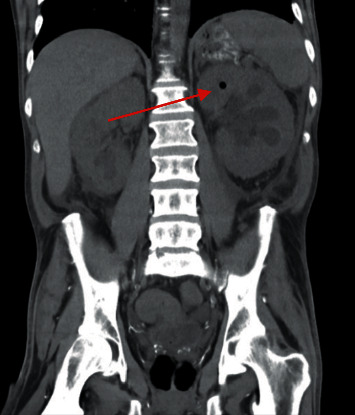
Coronal CT image showing evidence of diminished emphysematous pyelonephritis (red arrow) compared to Figure 1.
